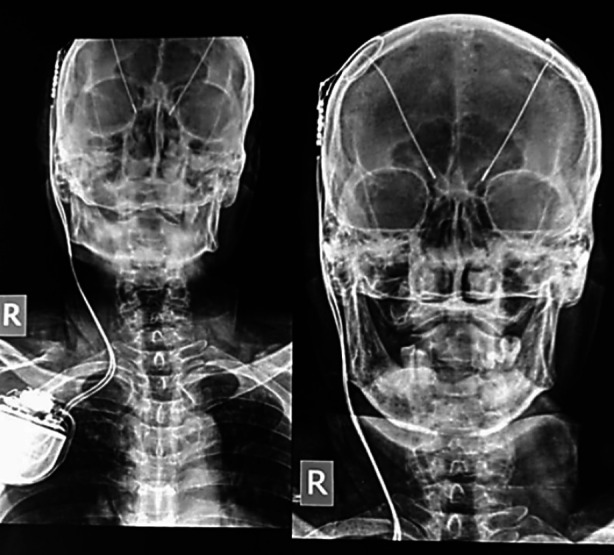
Showing post DBS x-ray. We can appreciate leads and Internal pulse generator (with patient’s permission).
